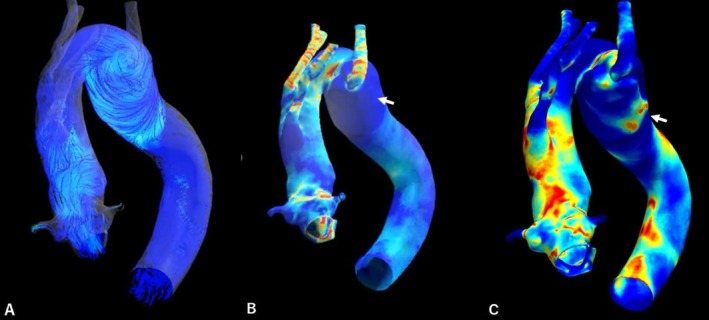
Computational fluid dynamics (CFD) analysis in Case 1. CFD analysis was performed on a pre‐dissection model reconstructed from CT data. (A) Blood flow velocity streamlines reveal a large vortex within the Kommerell's diverticulum (KD). (B) Wall shear stress (WSS) map exhibits diffusely low WSS (blue areas). (C) Oscillatory shear index (OSI) map. The white arrow indicates a region of high OSI on the posterior‐to‐inferior wall of the KD, which corresponded closely to the actual site of the dissection entry tear.
